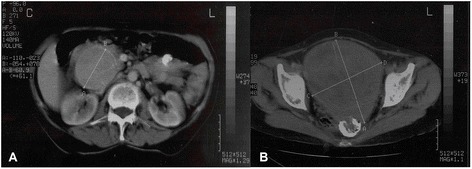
a CT image of retroperitoneal tumor located under the liver. b CT image of pelvic formation
